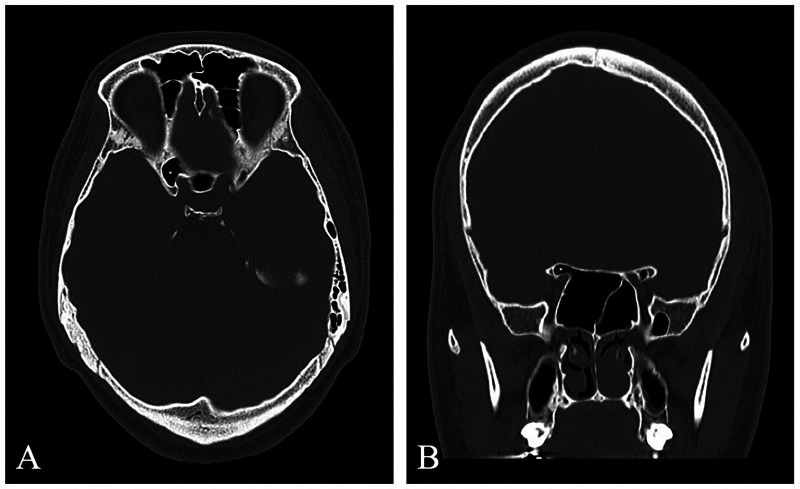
Bilateral type 2 anterior clinoid process (ACP) pneumatization. (A) Axial CT image showing left ACP pneumatization via the optic strut with ≤50% involvement (Type 2a, arrow) and right ACP pneumatization via the optic strut with >50% involvement (Type 2b, asterisk). (B) Coronal CT image of the same patient confirming left Type 2a and right Type 2b ACP pneumatization.
